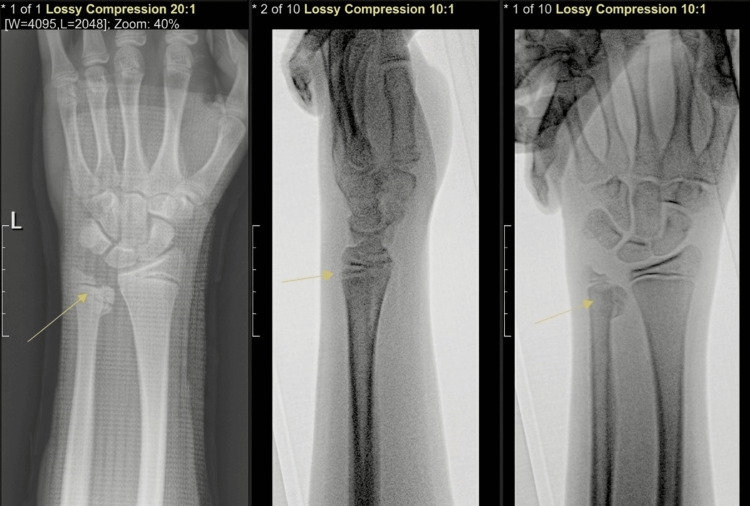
Radiographs obtained at the initial emergency department presentation Preoperative radiographs of the left wrist anteroposterior/posteroanterior (AP/PA; left panel), lateral (middle panel), and oblique (right panel), demonstrating a distal ulnar physeal fracture consistent with a Salter Harris IV (SHIV) fracture pattern (Day 0).
